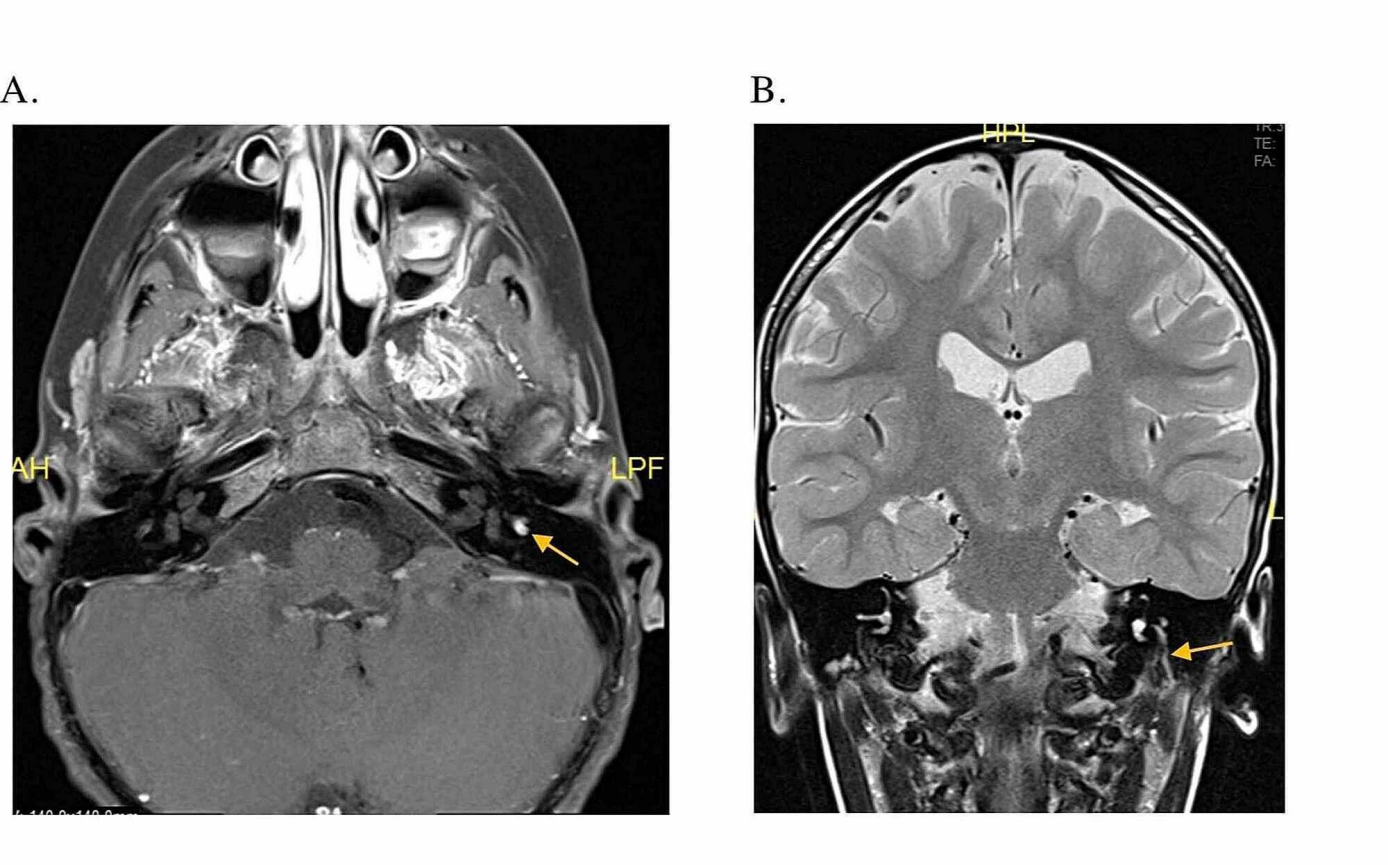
High-resolution MRI of the internal auditory canal. A. Axial T1 post-contrast MRI showing enhancement of the left facial nerve in the geniculate ganglion. B. Coronal T1 post-contrast MRI shows enhancement of the left seventh cranial nerve from the level of the geniculate ganglion all the way down to the stylomastoid foramen. The descending mastoid segment of the facial nerve on the left appears significantly larger than the nerve on the right.
